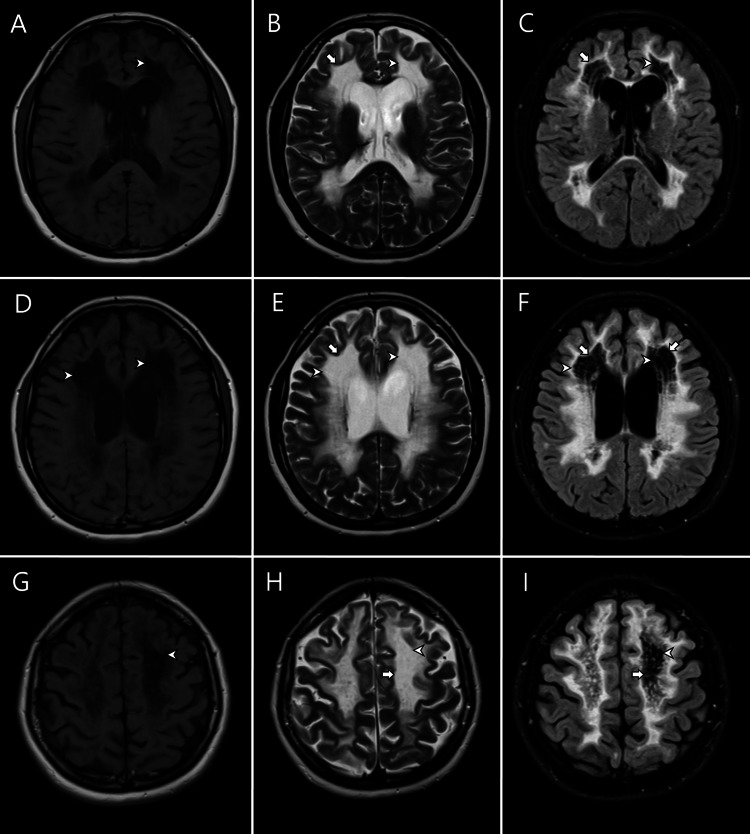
Brain MRI Axial MRI showing bilateral diffuse white matter affection that appears hypointense on T1-weighted magnetic resonance (MR) images (panels A, D, and G) and hyperintense on T2-weighted (panels B, E, and H) and T2-fluid-attenuated inversion recovery (FLAIR) MR images (panels C, F, and I) compared to normal white matter. Also, some lesions isointense to CSF at T1-weighted, T2-weighted, and FLAIR images representing cystic degeneration of white matter can be seen (arrowheads).
